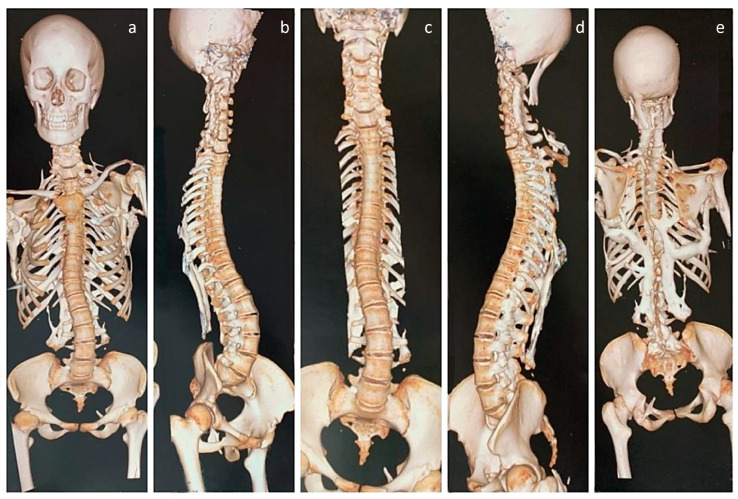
Three-dimensional CT images of the upper body, pelvis, and hip joints of an 18-year-old female with a clinicoradiological diagnosis of fibrodysplasia ossificans progressiva (FOP), an exceptionally rare genetic disease affecting 1 in 2 million people that is caused by a gain-of-function mutation in the gene that encodes activin A receptor type I (ACVR1) [1,2]. The disease is characterized by progressive heterotopic ossifications in various extraskeletal sites [3]. In the patient reported here, the disease initially presented as neck stiffness, followed by multiple episodes of back stiffness, the appearance of notable hard lumps, and finally, restricted movement in the right shoulder joint, with pain preceding stiffness in all affected locations. (a–d) Thoracolumbar scoliosis and complete ossification of the disc spaces in the thoracic spine can be noted in both the anterior and oblique views, while fusion of the posterior elements, especially at the lumbar region, is evident on the oblique views. (b,d) The cervical spine appears to be fused, as indicated by fused lateral masses and posterior elements. (d,e) Heterotopic bone is observed, descending from the occiput, potentially indicating an ossified capitus muscle on the left side. (e) On the posterior aspect, a large mass of heterotopic bone is observed, corresponding to the location of the paraspinal muscles, branching upwards and fusing with the scapulae on both sides. On the right side, the heterotopic bone mass is fused with the humerus. (a,e) In addition, small, heterotopic bony projections are seen, originating from the first rib and pointing upwards. The rest of the upper limb was unaffected, allowing for full active range of motion in both elbows, wrists, and hands. Physical function, as measured by the SF-36 score, showed significant impairment (20/100), which affected her work and daily activities. As a result, her emotional and social well-being were also impacted. There were no obvious soft-tissue flare-ups at the time of presentation; however, she had hip pain that was described as moderate and interfering with daily activities.
